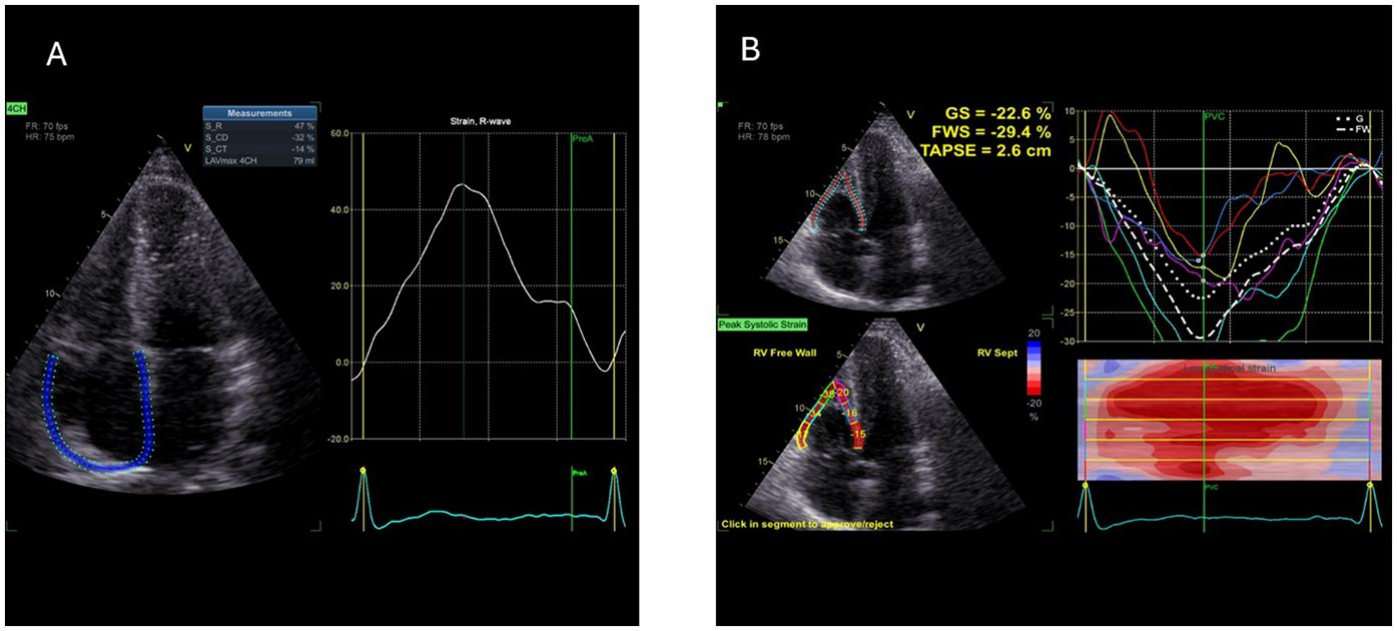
Example of 2D speckle tracking examination of right atrial (A) and right ventricular (B) strain—picture taken from control sample (2D ST, 2-dimentional speckle tracking; CD, conduit; CT, contraction; GS, global strain; FWS, free wall strain, R, reservoir; TAPSE, tricuspid anulus plane excursion).
